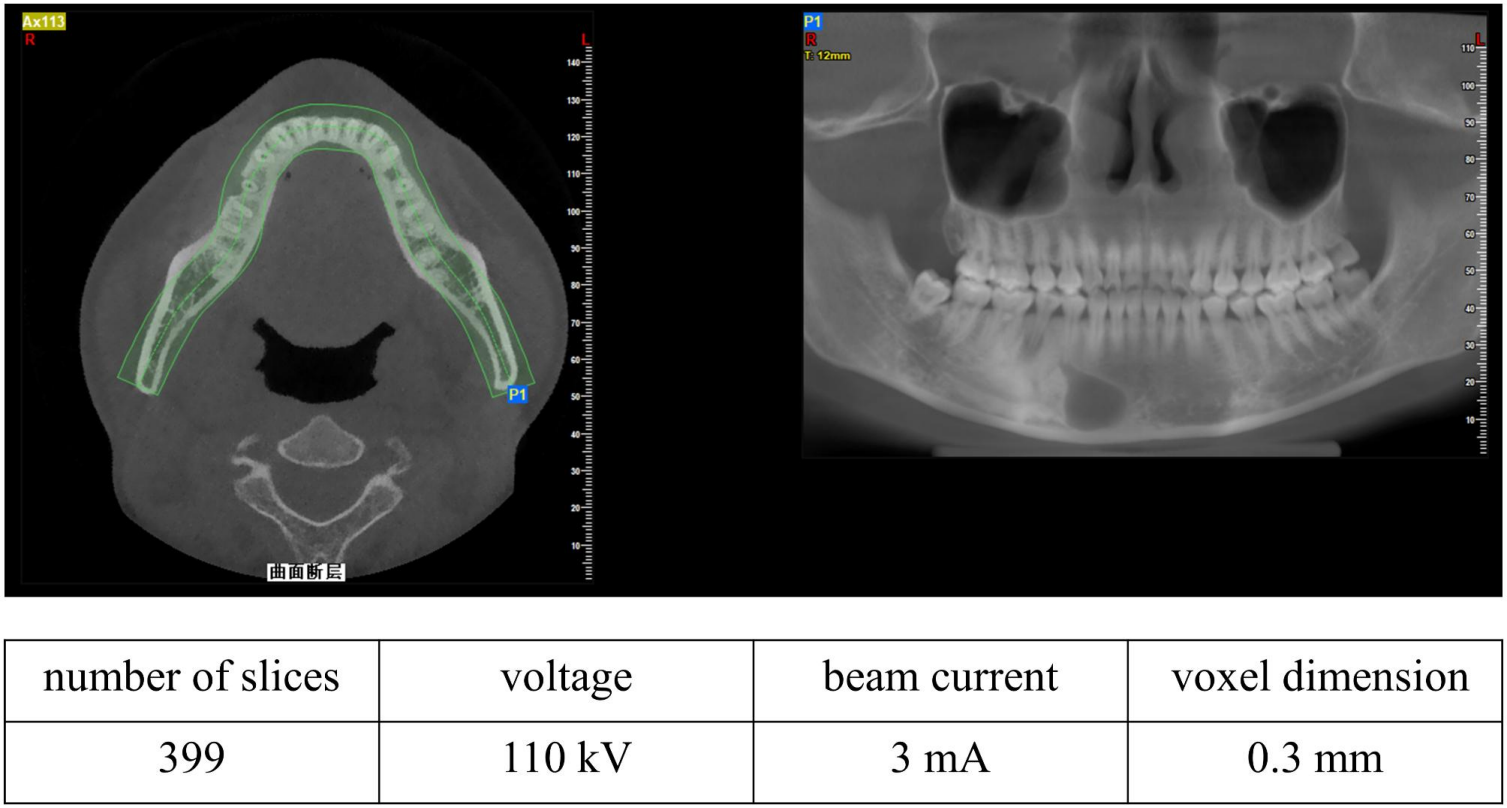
Typical CBCT images of periapical cysts with corresponding parameters
